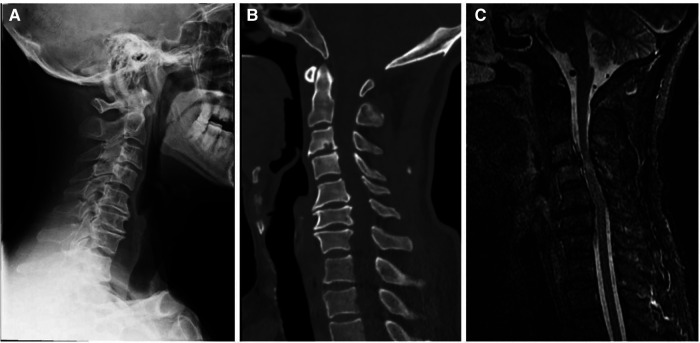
Preoperative x-ray (A), CT (B), and MRI (C) showed a diagnosis of traumatic cervical myelopathy.
